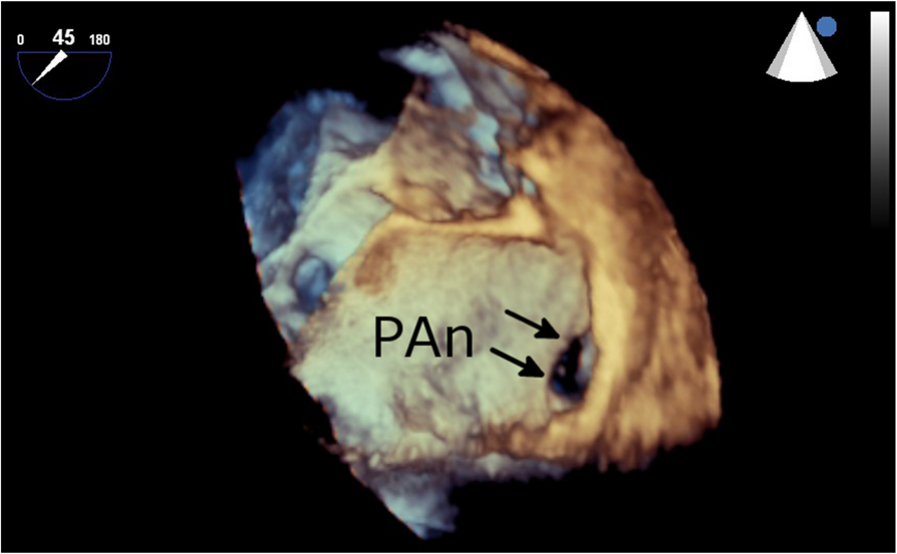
Three-dimensional transesophageal echocardiography reveals the pseudoaneurysm and the neck
